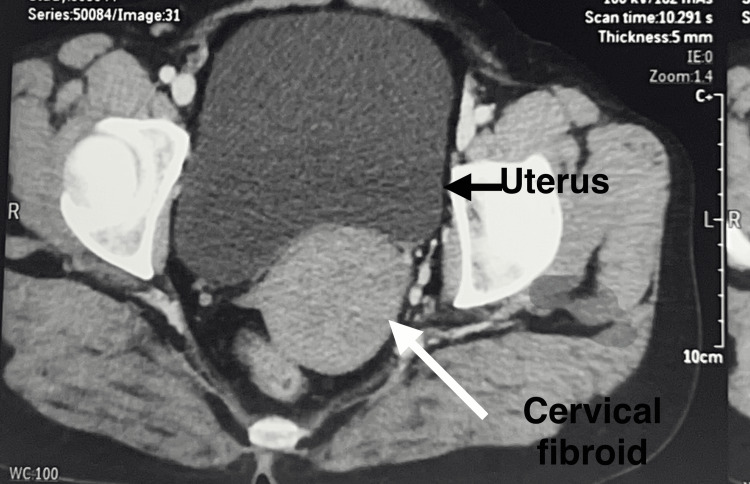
Contrast-enhanced CT of the abdomen and pelvis revealed a cervical fibroid measuring 6 x 7 cm
